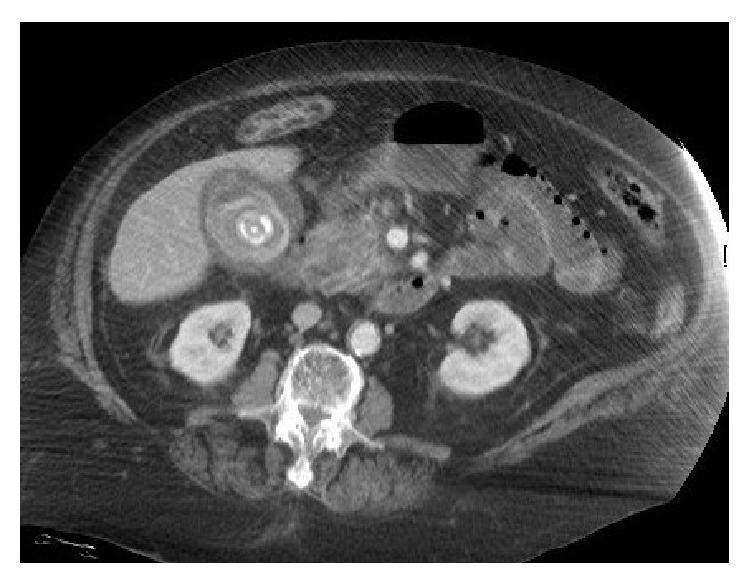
Axial CT image demonstrating gallbladder wall thickening, mucosal hyperenhancement, and a calcified gallstone.
